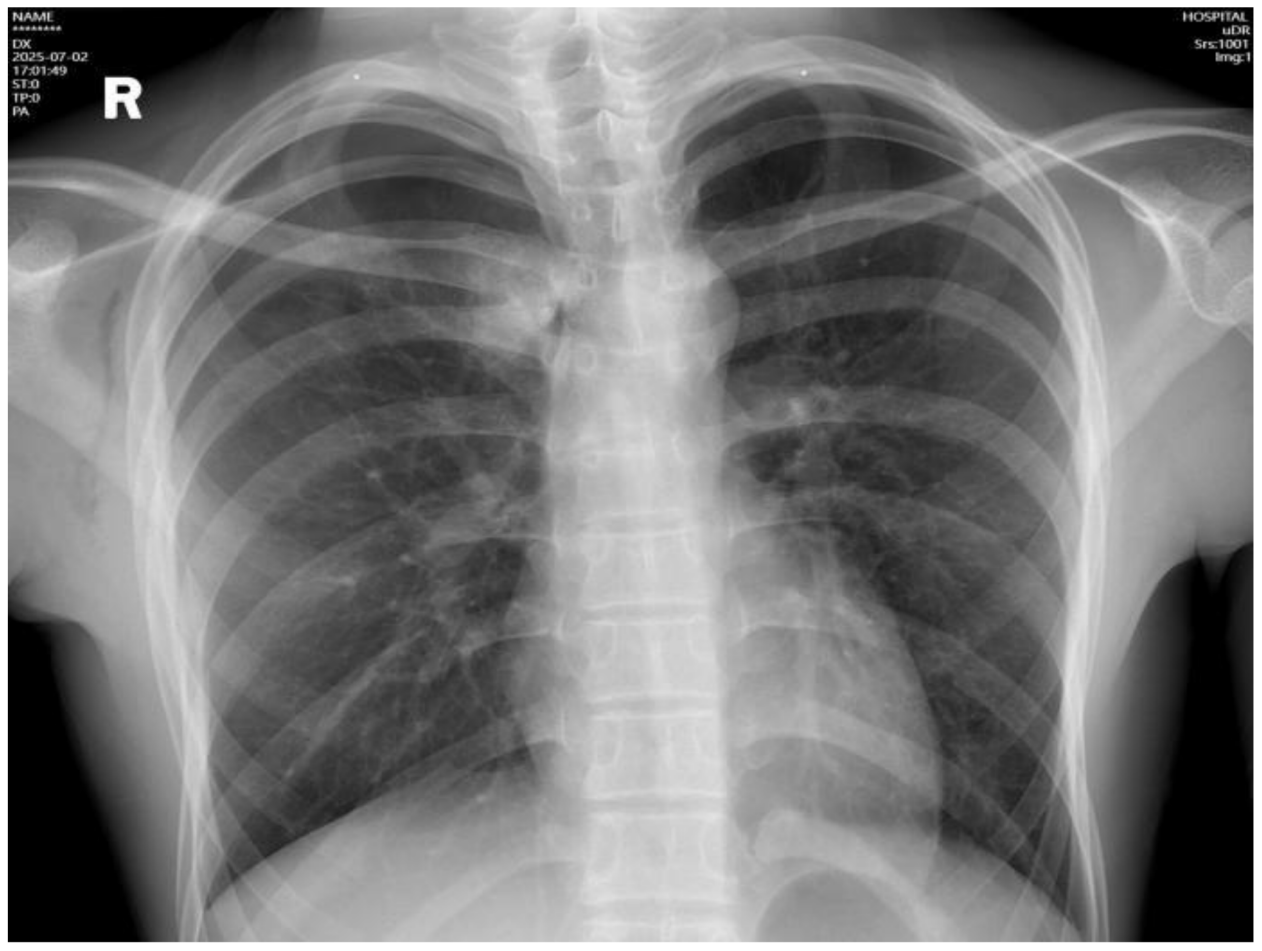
Postoperative chest X-ray demonstrating satisfactory lung recruitment.
